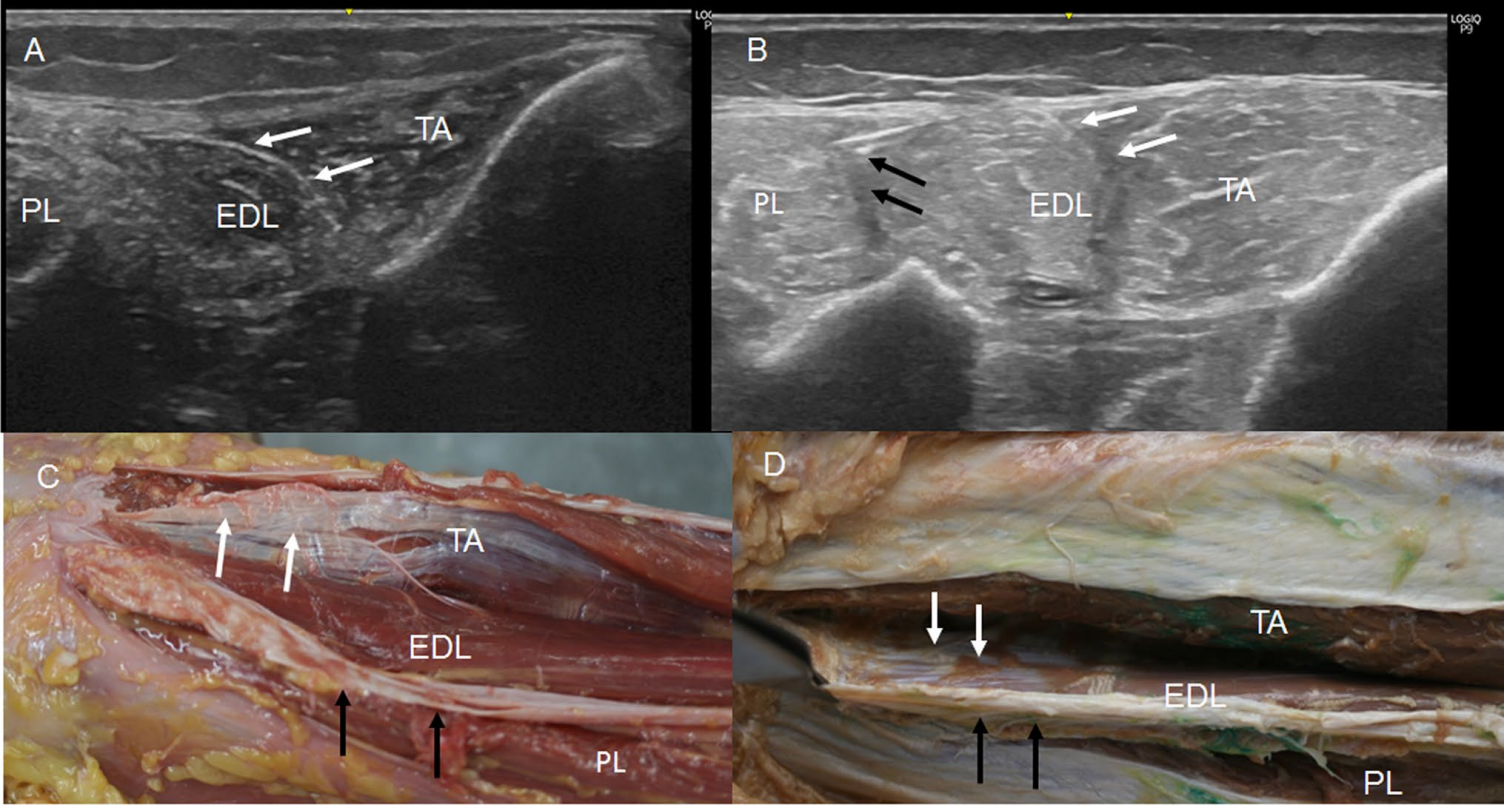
Ultrasound image of the septum between the tibialis anterior muscle (TA) and extensor digitorum longus muscle (EDL) A a hyperechoic line (white arrows) B a hypoechogenicity line (white arrows) between TA and EDL. Black arrows show the septum between the EDL and peroneus longus muscle (PL) C The dissection shows this septum (white arrows) as an aponeurosis that belongs to the TA. Black arrows show the septum between the EDL and PL . D It shows the aponeurosis that corresponds to the EDL. Black arrows show the septum between EDL and PL
